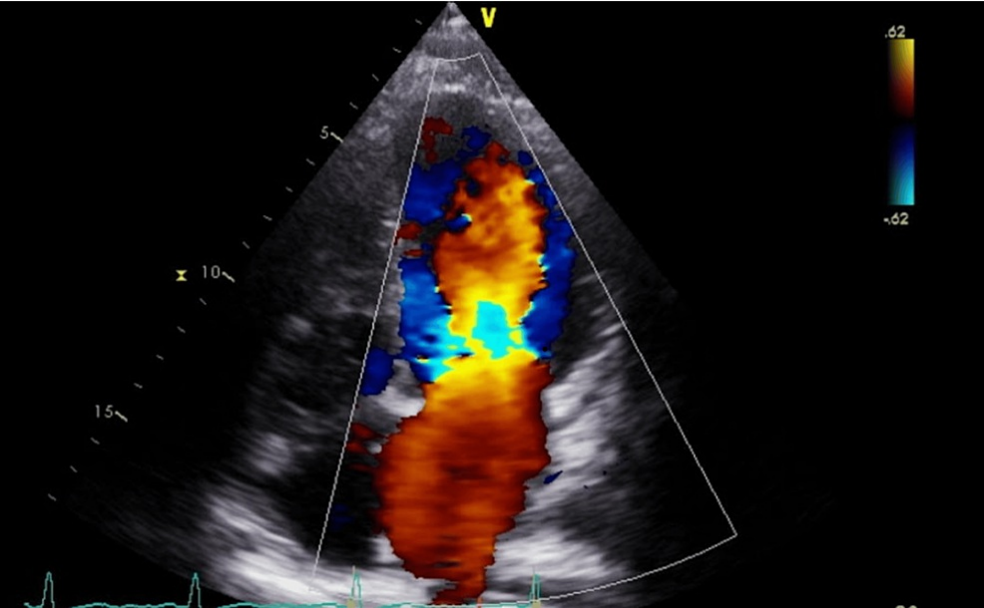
Echocardiogram Doppler visually demonstrates moderate to severe mitral regurgitation
